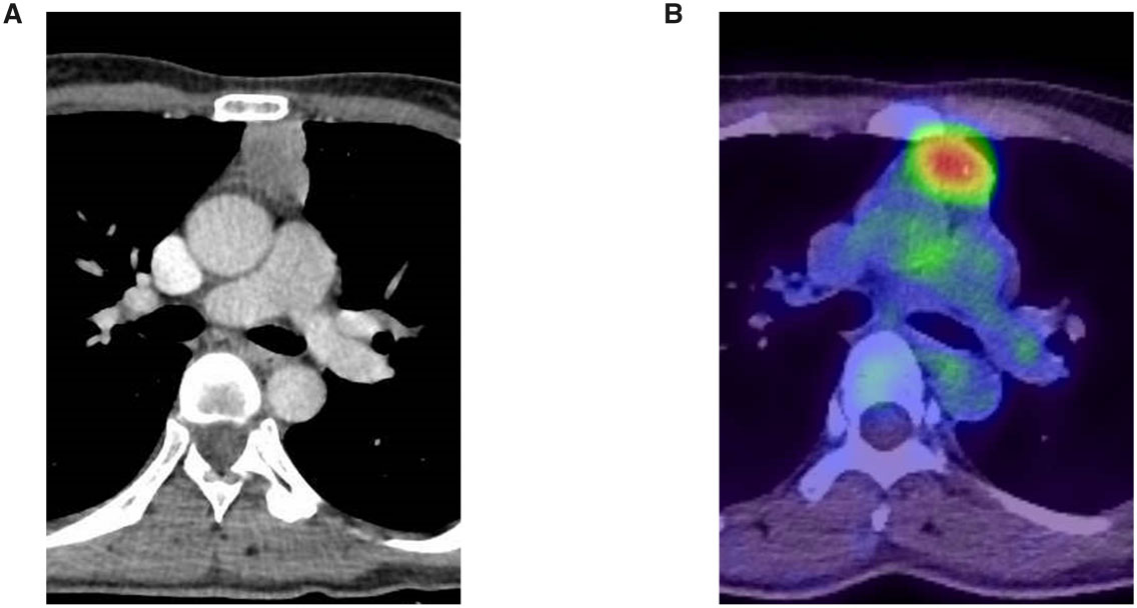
Representative images of clinical stage I type B3 thymoma that required combined resection of the innominate vein: (A) axial enhanced CT demonstrated that the maximum tumour diameter was 3.6 cm. (B) 18F-FDG PET/CT image shows high FDG uptake within the mass with SUVmax of 5.6. CT: computed tomography; 18F-FDG PET: fluorine-18-fluorodeoxyglucose positron emission tomography; SUVmax: maximum standardized uptake value.
